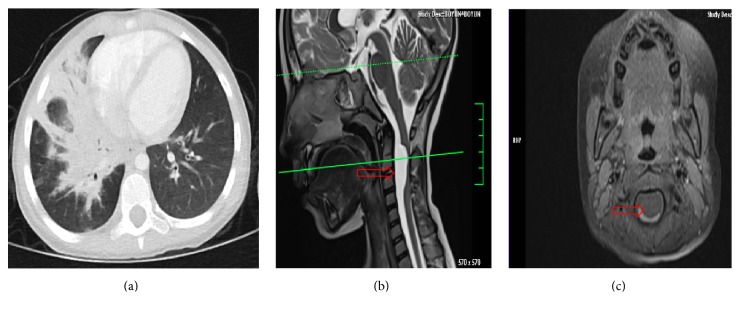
Thorax CT (b) and neck MR (b-c): right lung consolidation and cervical spine arachnoid cyst (arrow).
